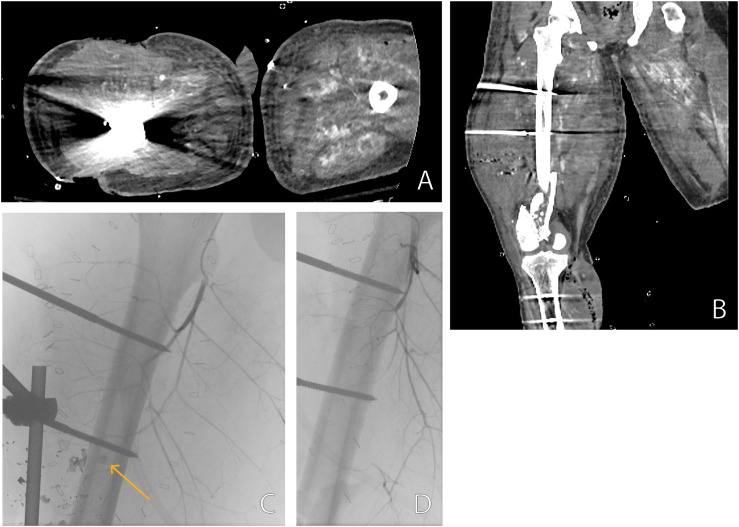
(A-D). Exemplary case of late vascular injury. Axial (A) and coronal (B) CTA of a 22 years old soldier who suffered multiple gunshot wounds, performed one month after the initial injury. Artifacts from external fixation of the femur and signs of rhabdomyolysis such as mild fiber-like enhancement after contrast administration and skeletal muscle calcification are visible, obscuring vascular structures and possible pathologies. Image C shows a same day angiography of the same limb, revealing a pseudoaneurysm of a PFA branch (arrow). The leading artery was embolized with Concerto Detachable Coil, image D shows the post procedure resolution of the pseudoaneurysm.
